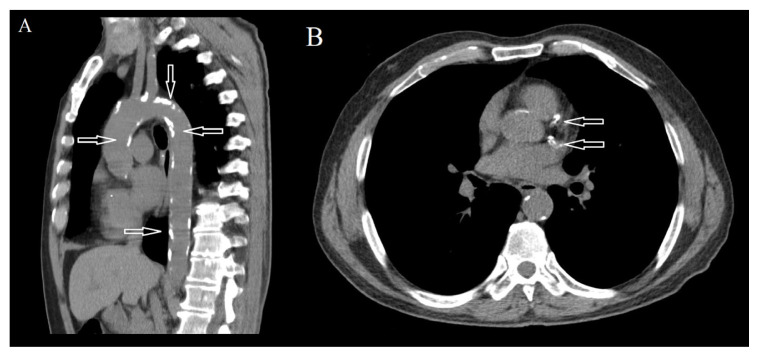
59-year-old man with gastric wall fat halo sign. Unenhanced sagittal CT image shows extensive calcific atherosclerotic plaques in aorta (A) and unenhanced axial CT image shows extensive calcific atherosclerotic plaques in coronary arteries (B).
